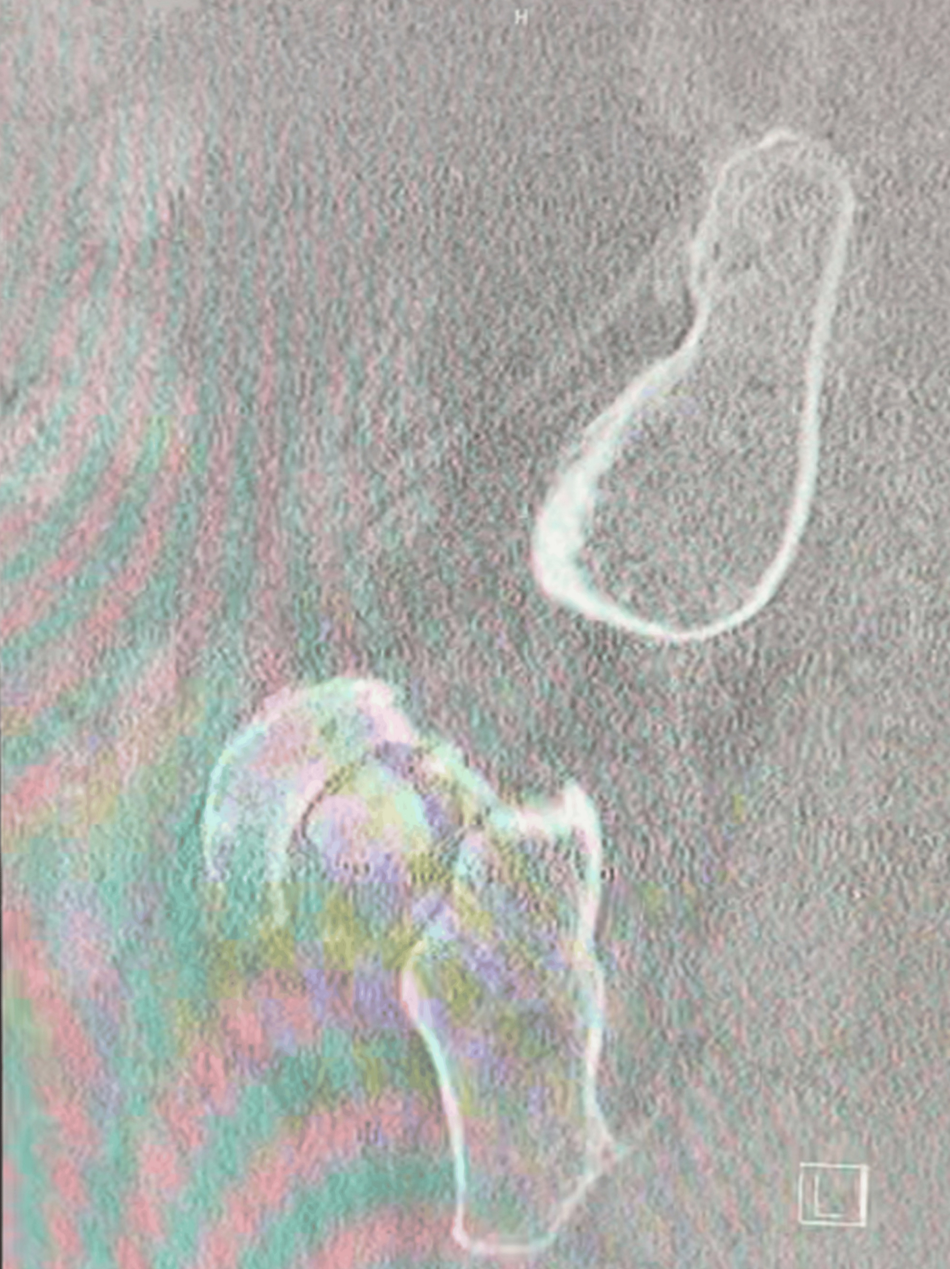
Additional preoperative image of sagittal CT left hip
